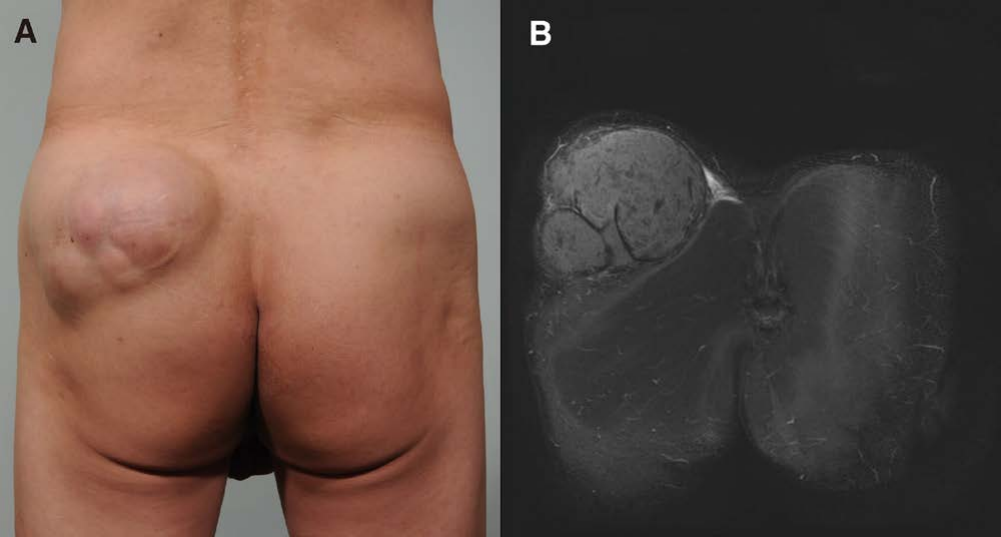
(A) Case 7: The largest MGEC in this study, located on the left buttock (10 × 7 × 6 cm^3^). Multiple lobules are observed on the surface. (B) MR images demonstrating hyperintense STIR signal intensity lesion with multiple lobules. MGEC = multilocular giant epidermal cyst, STIR = short tau inversion recovery.
